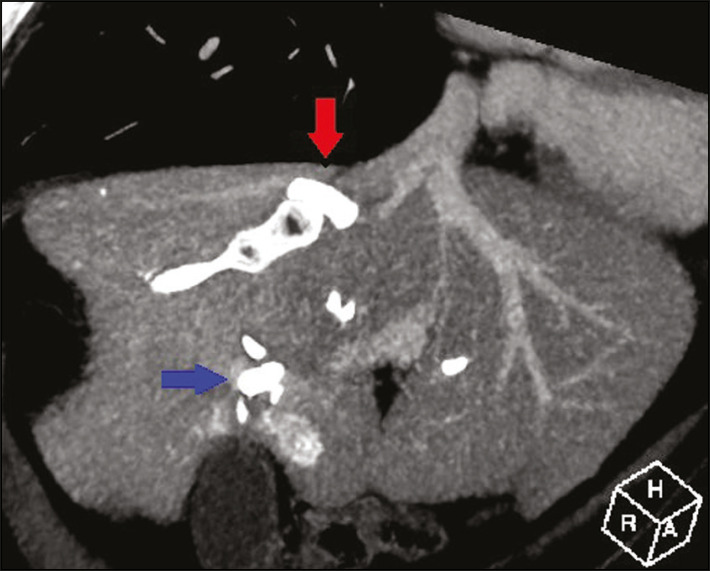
Reconstruction of contrast-enhanced oblique coronal venous phase CT scan, acquired after LVD, showing the RHV embolized with the vascular plug (red arrow) and some portal branches embolized with Histoacryl and Lipiodol (blue arrow).
